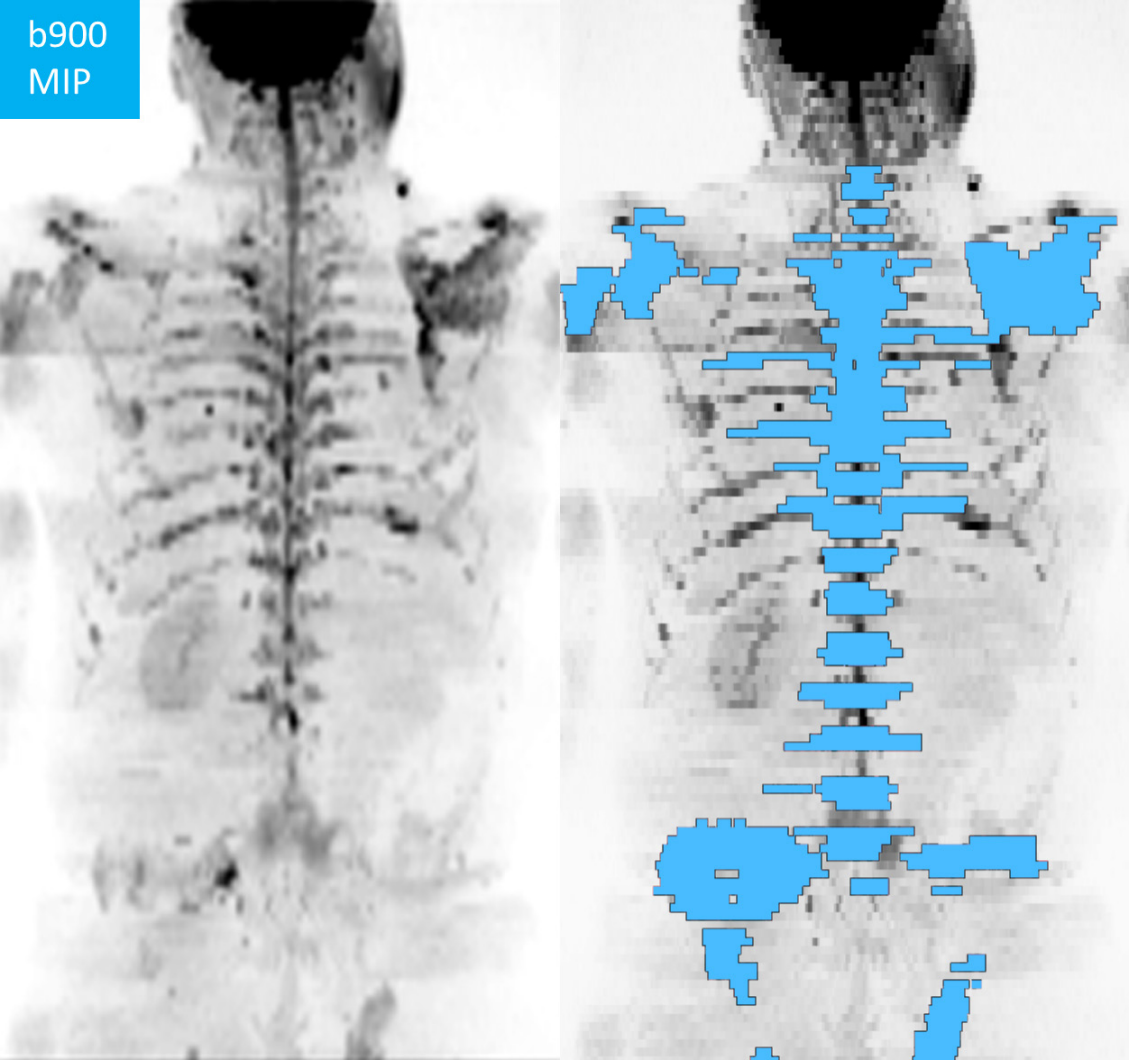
Total bone disease volume segmentation in a 61-year-old metastatic prostate cancer patient, DWI b900 maximum intensity projection (MIP) with superimposed disease volumes of interest (blue).
